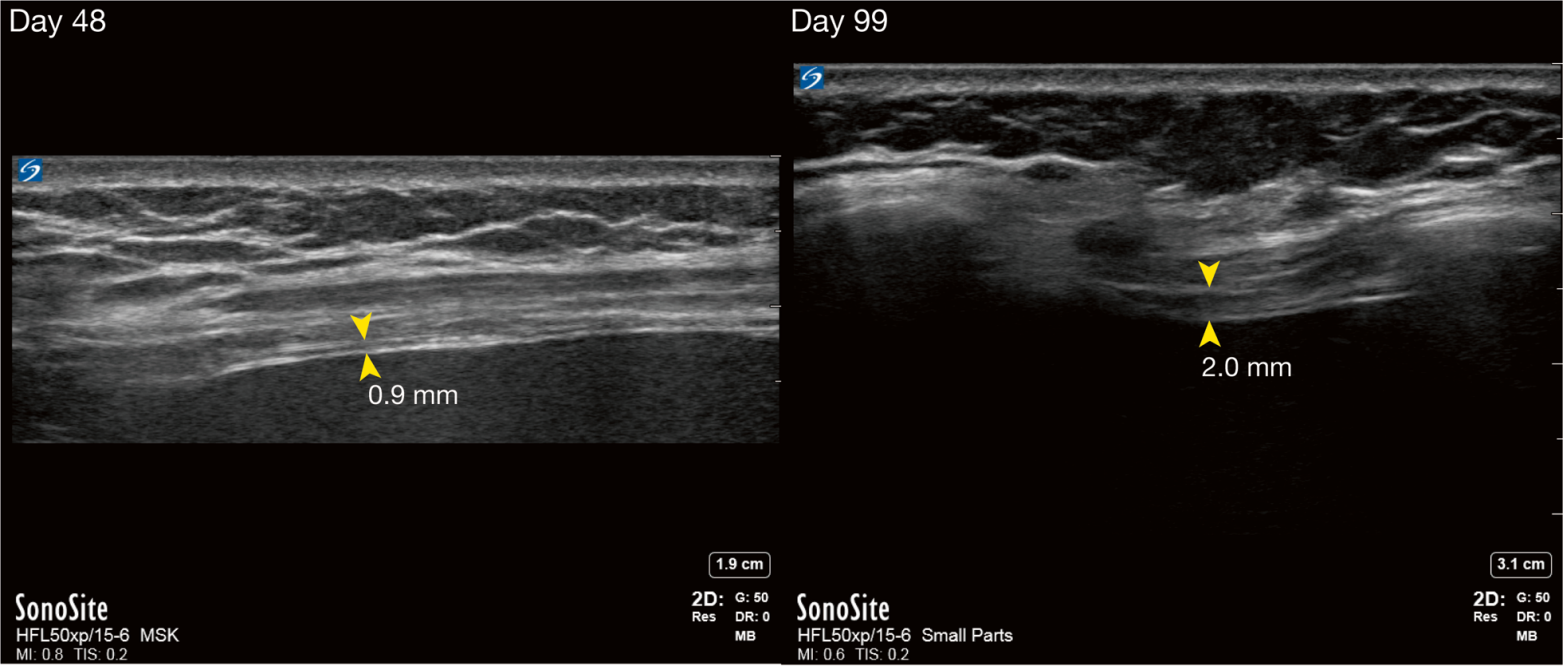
Images of diaphragm ultrasound at the zone of apposition of the right hemidiaphragm on day 48 (left) and day 99 (right). The thicknesses at the end inspiration are shown (arrowheads)
